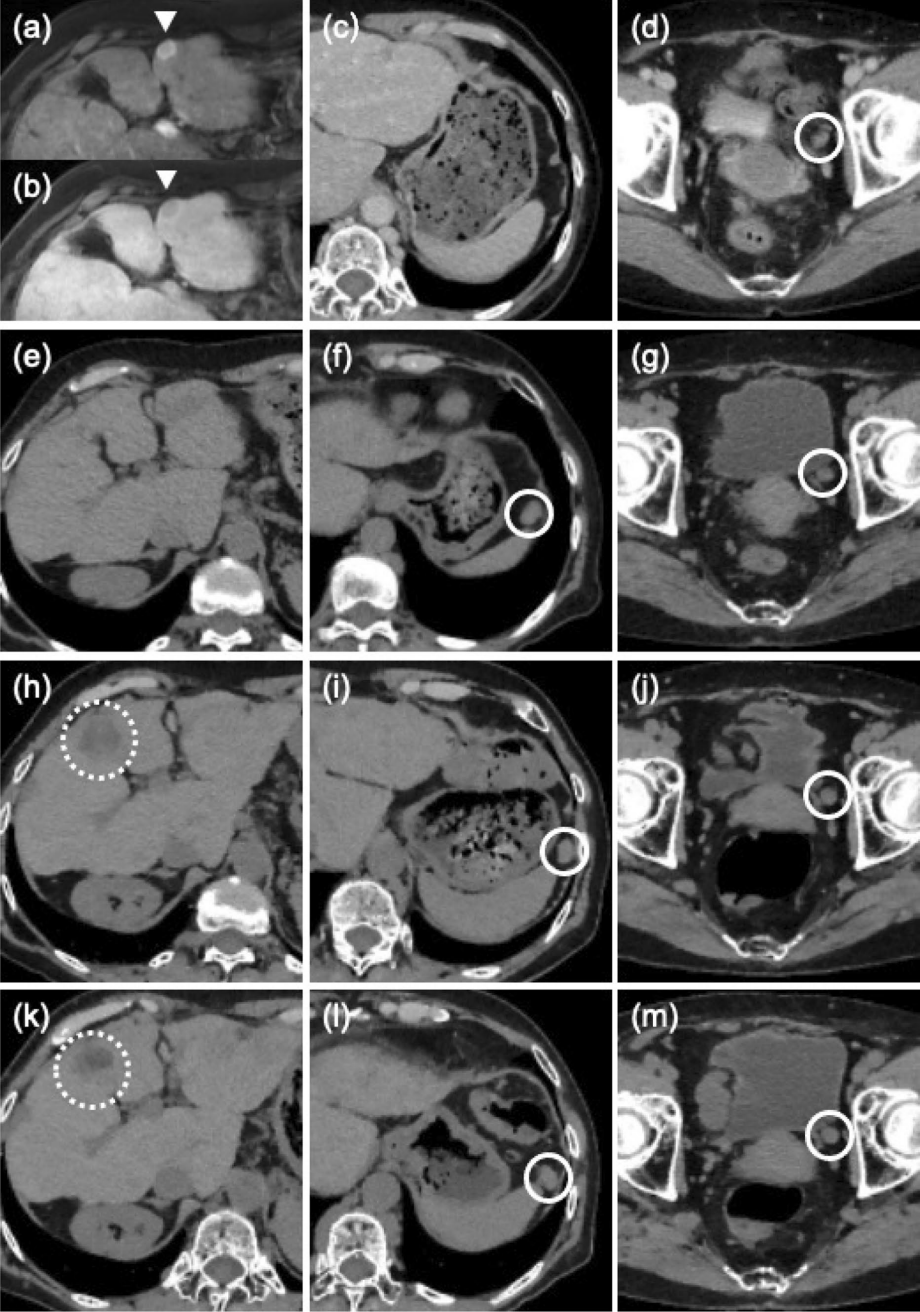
Representative images of the liver and abdomen after bilateral salpingo-oophorectomy. Intrahepatic recurrence was detected in 2012. Dynamic MRI showed early enhancement and wash out in early and delayed phase (arrow heads in a, b). Abdominal dissemination was indicated in the pelvis (d) and no nodule was near the spleen (c). Before administration of low-dose sorafenib in 2016 (e, f, g), a new disseminated lesion appeared near the spleen. Use of the contrast medium was avoided due to allergic reaction. Before percutaneous ethanol injection therapy (PEIT) in 2019, another intrahepatic lesion in S5 was clarified even in plain CT (h). The lesions of the abdominal dissemination were stable (i, j). After PEIT in 2020, The intrahepatic lesion in S5 include low density area which allowed to suggest necrosis (k). The lesions of the abdominal dissemination were stable (l, m). White solid and dotted circles show lesions of abdominal dissemination and an intrahepatic lesion in S5
